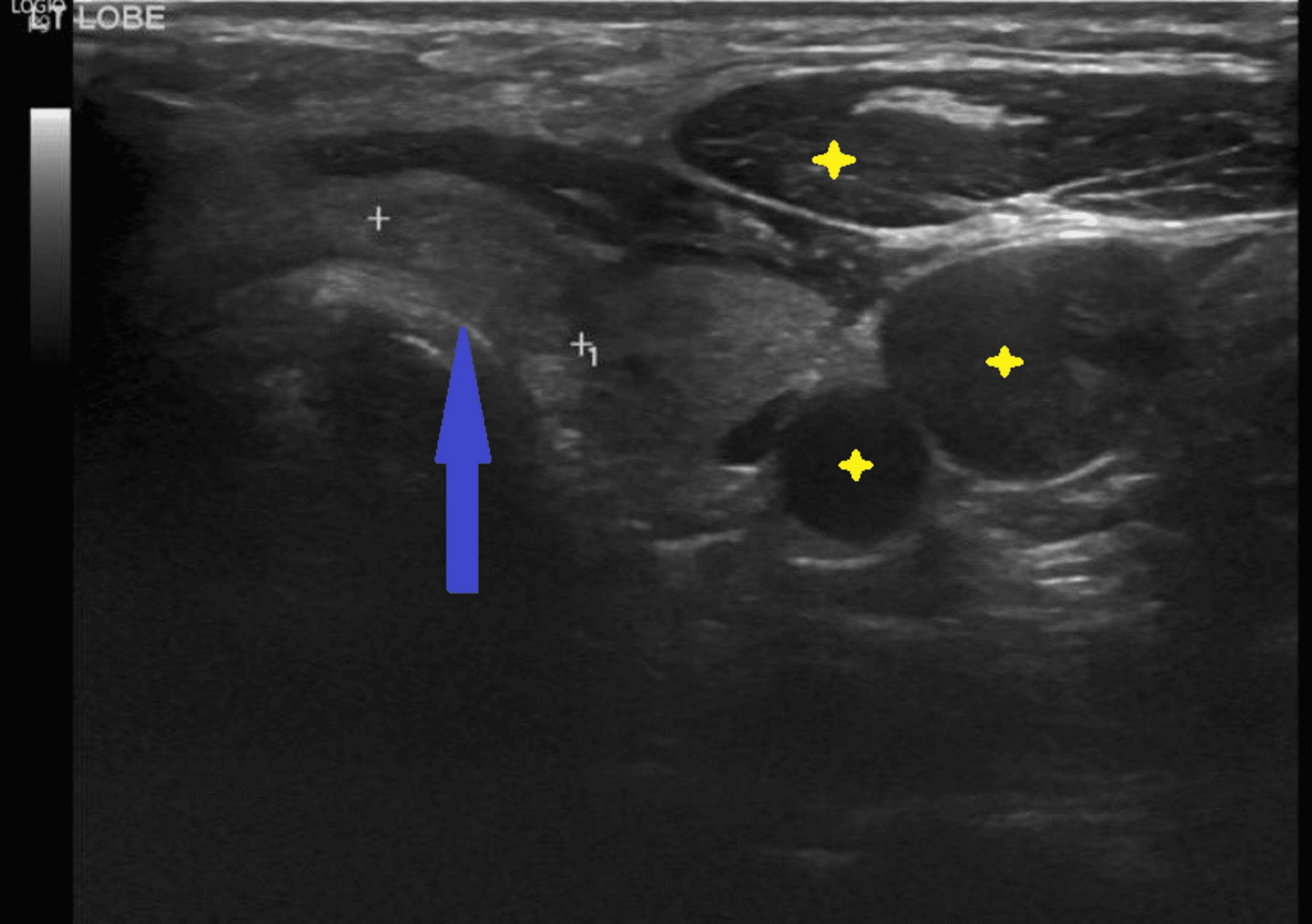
Uultrasound of the thyroid There are few small left thyroid lobe colloid cysts. The largest measured 7 mm (labelled by yellow stars), and the small, well-defined, solid isoechoic left isthmic junction measured 11x4 mm with no calcifications (pointed by a blue arrow). Thyroid Imaging Reporting and Data Systems (TIRADS)-3.
